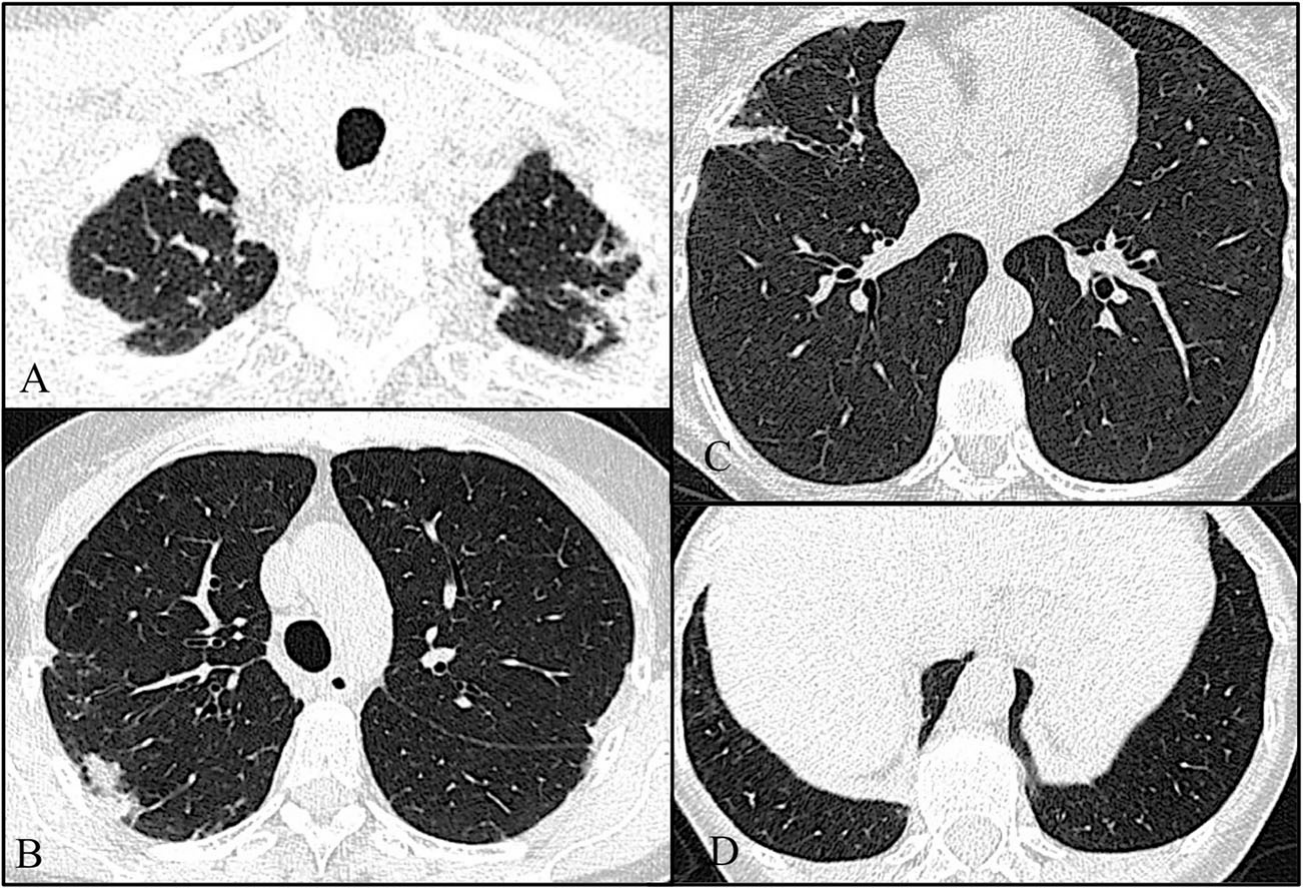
Axial high-resolution chest tomography (HRCT) images (a–d) of patient 4 show pleural and subpleural thickening with moderate fibrotic changes in the marginal parenchyma with apical-caudal distribution. These features are more evident in the right lobe. Note also, in c, a slight “tree-in-bud” pattern in the middle lobe compatible with concomitant pulmonary infection
